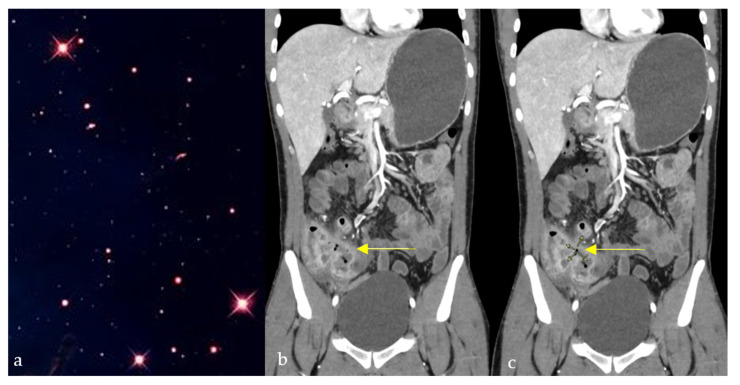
Coronal CT images of the abdomen and pelvis showing a conglomerate of inflamed bowel loops interconnected by multiple fistulous tracts (b,c), giving a star-shaped configuration (yellow arrows) resembling its natural counterpart (a).
